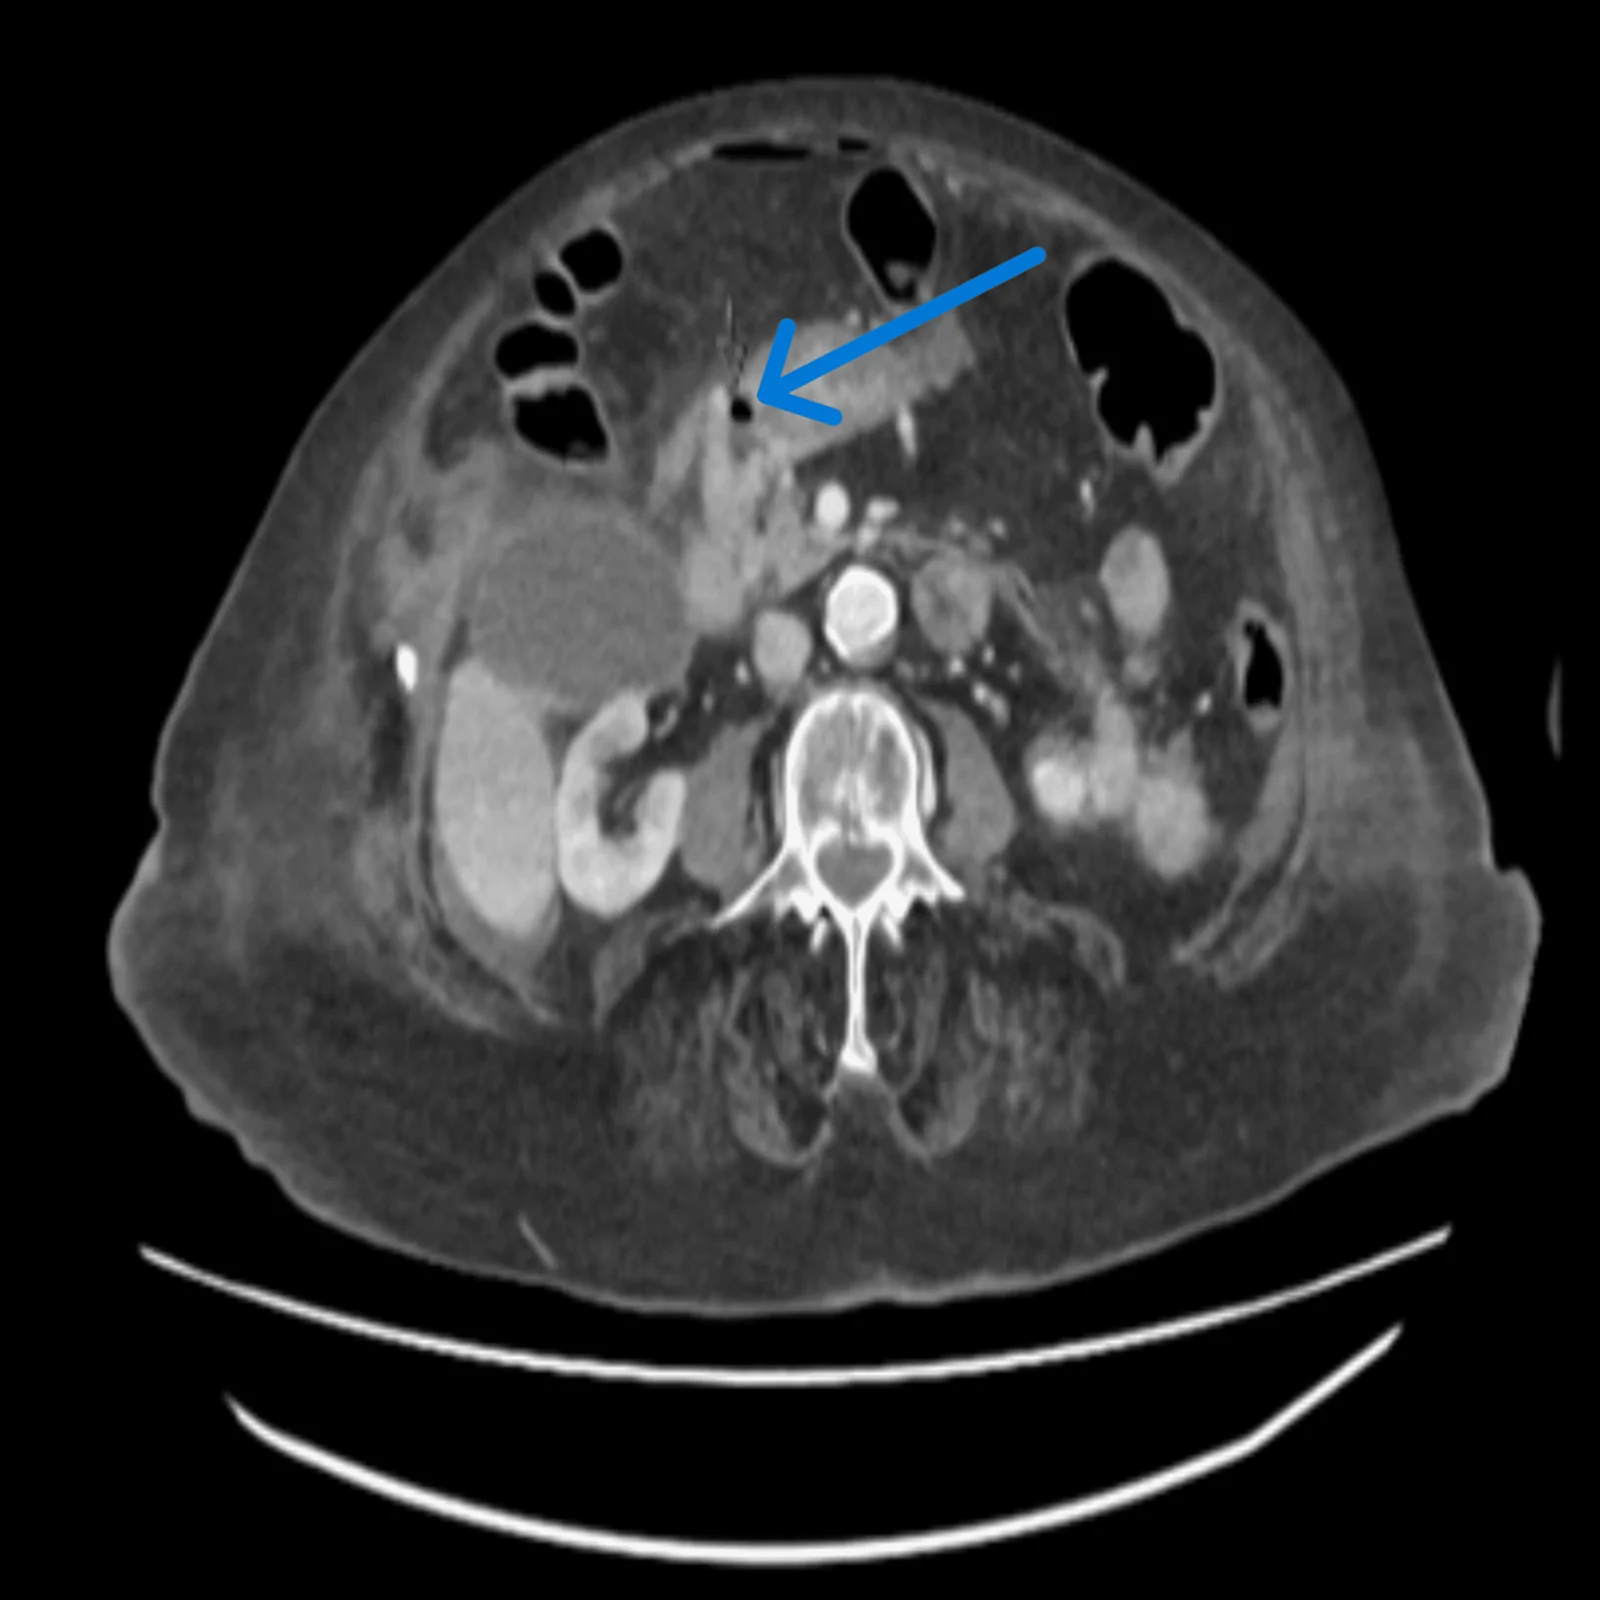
Computed tomography of the abdomen (non-contrast) The arrow demonstrates a perforation at the gastric wall.
